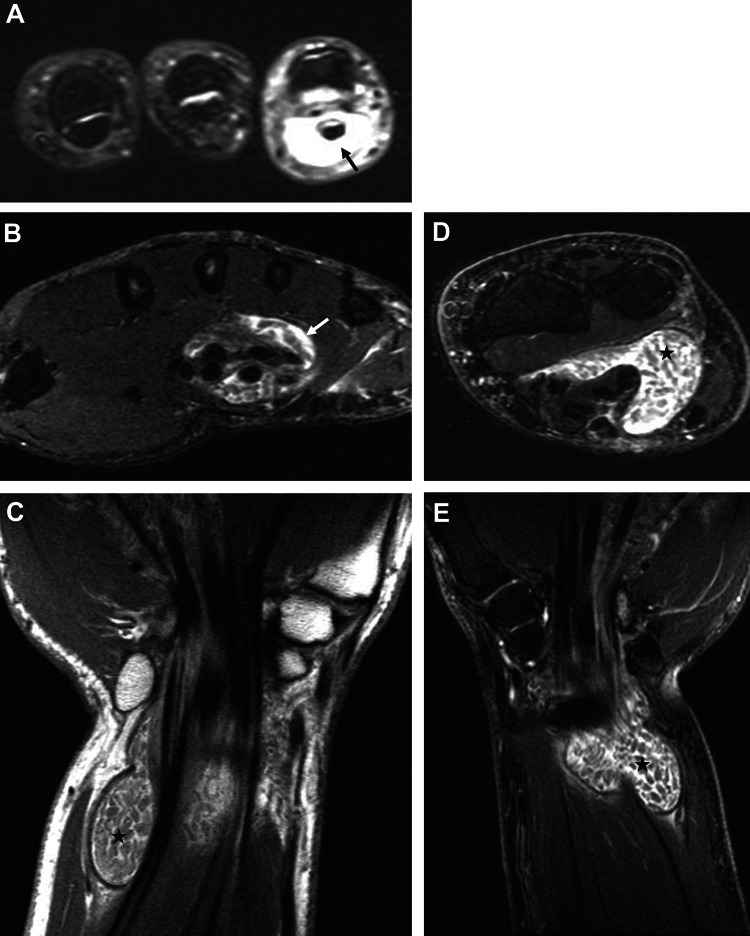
Preoperative MRI of the left hand, wrist, and distal forearm In the axial image of the fingers (A), flexor tenosynovitis of the left small finger is highlighted by a black arrow. Moving to the axial image of the hand at the level of metacarpal bones (B), both flexor tenosynovitis and synovial hypertrophy are evident, indicated by a white arrow. The axial (D) and coronal (C and E) images of the left wrist and distal forearm show a loculated fluid in the volar aspect of the wrist, marked by black asterisks, containing rice bodies
